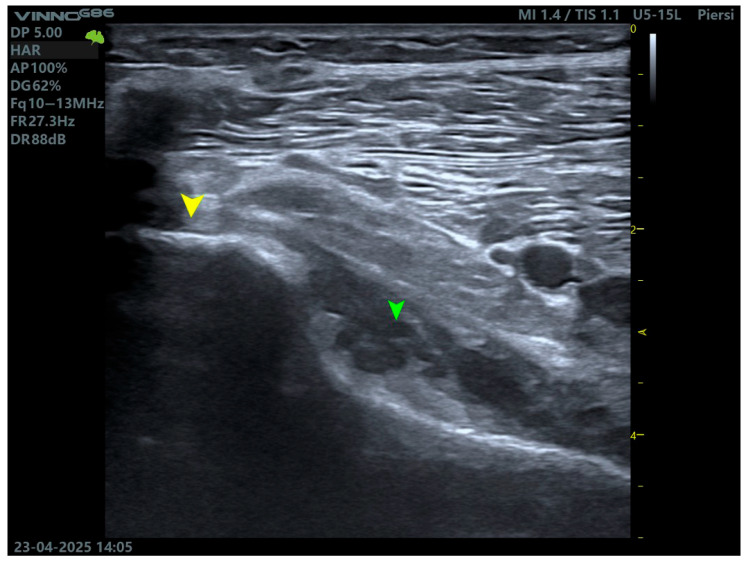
Ultrasound imaging of the popliteus muscle. The yellow arrow indicates the tendon, while the green arrow indicates the muscle belly.
